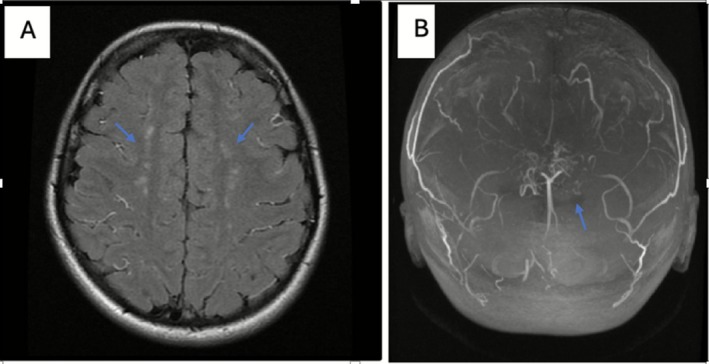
(A) MRI of the brain showed increased T2 and Flair signals foci in frontal subcortical and periventricular white matter. (B) MRA showing Narrowing of Anterior, Middle, and Posterior Cerebral Arteries.
